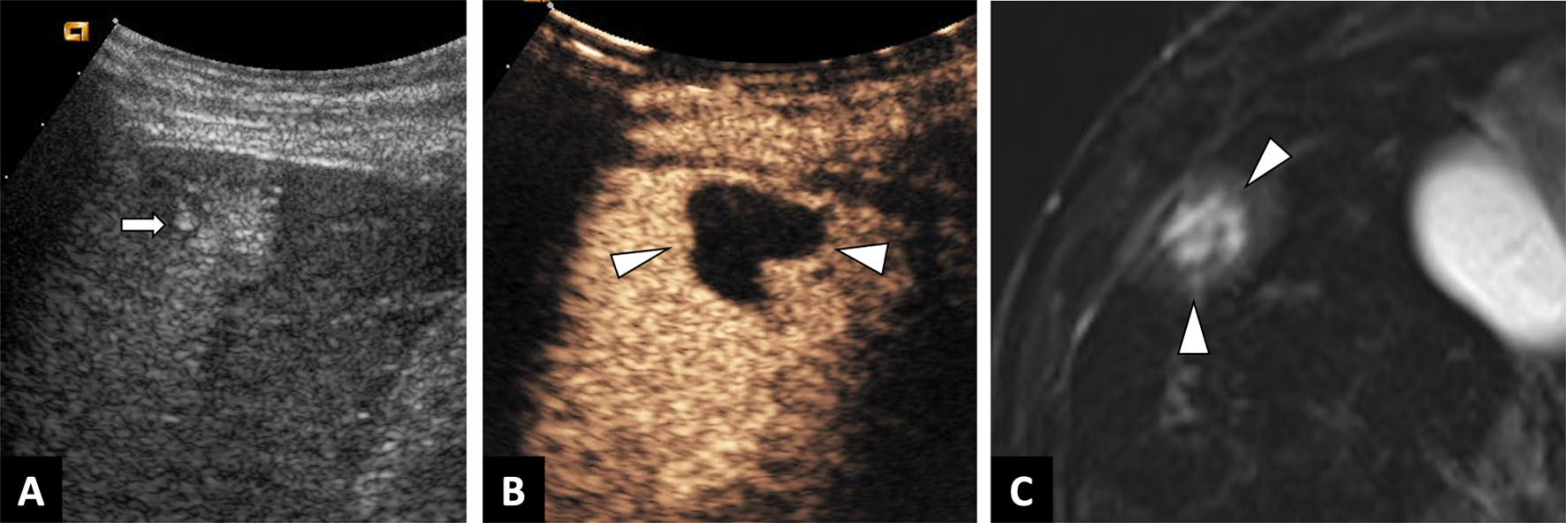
Incidental alveolar echinococcosis discovered in a 65-year-old man with a recent history of acute myeloid leukemia, and presenting with liver blood test abnormalities. A. Abdominal ultrasound showing heterogeneous echogenic mass with irregular borders. B. Contrast-enhanced ultrasound showing the absence of enhancement of the liver lesion (arrowheads). C. T2-weighted MR images in the axial plane showing pathognomonic microcysts (arrowheads)
